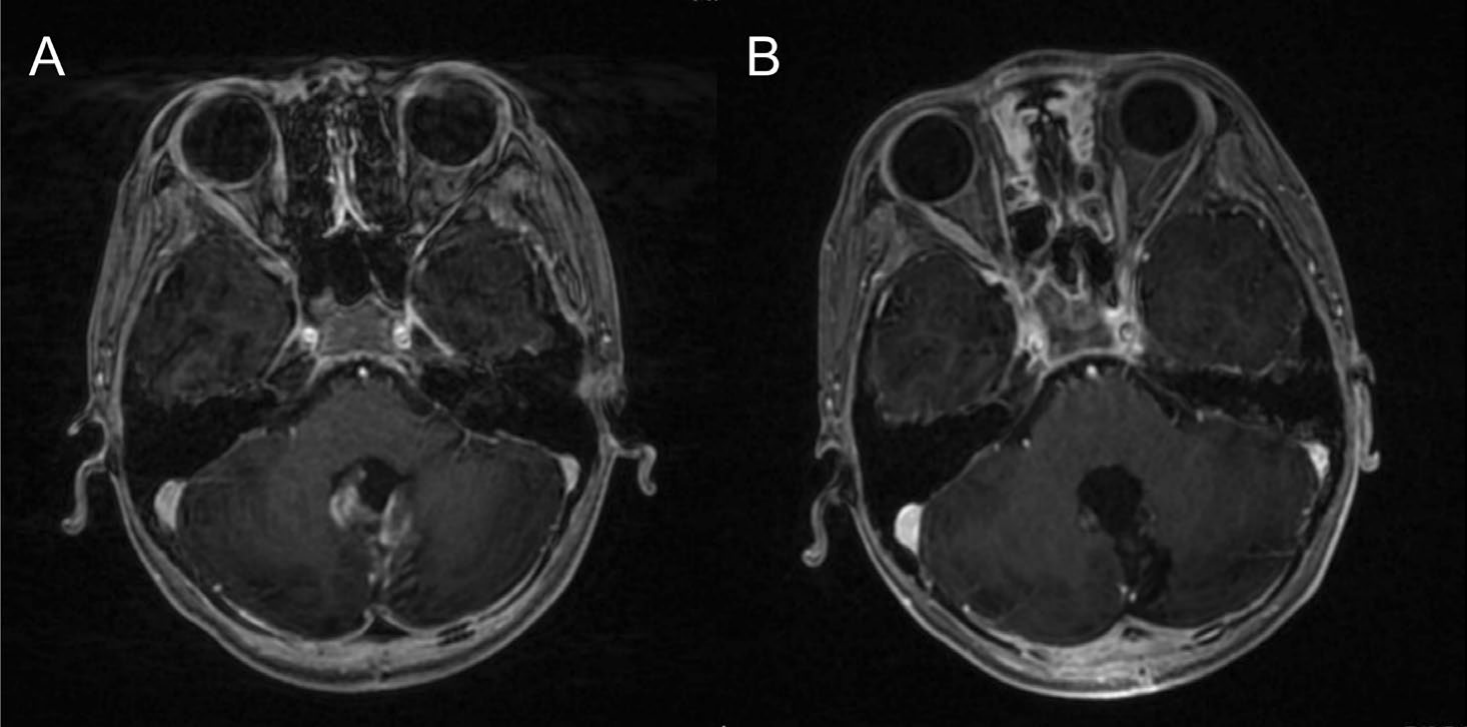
MRI images of a medulloblastoma patient before and after PBT. (A) Axial T1‐weighted MRI with contrast post‐surgery shows a residual medulloblastoma tumor in the posterior fossa region before PBT. The residual tumor appears as an enhanced mass adjacent to the brainstem. (B) Axial T1‐weighted MRI with contrast after PBT demonstrates a significant reduction in tumor size, with no visible enhancement in the treated area, indicating a CR to the therapy. MRI, magnetic resonance imaging; PBT, proton beam therapy; CR, complete response.
